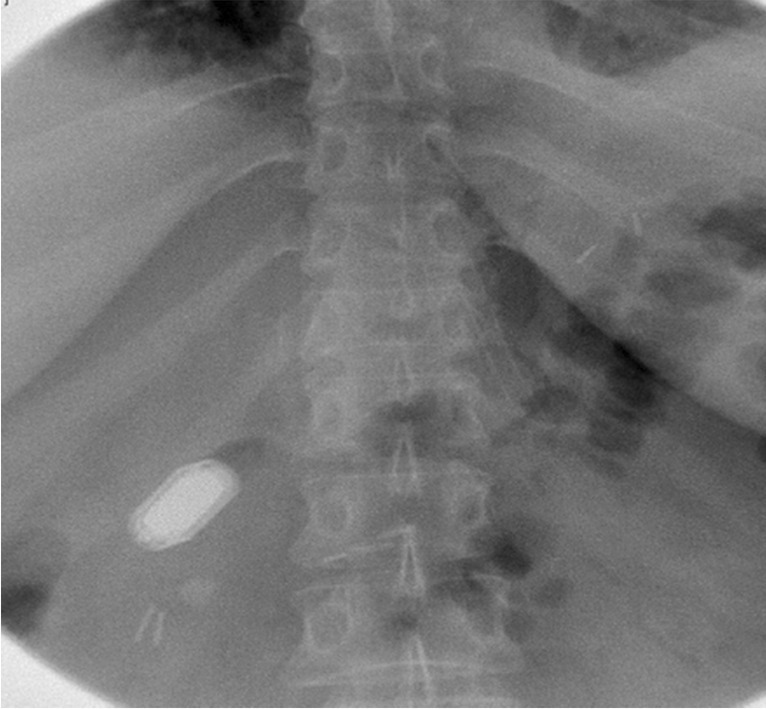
Patient radiograph of magnet alignment and fusion in the right upper quadrant, postoperative day 1. Note the presence of marking clips at 250 cm on the magnet side
